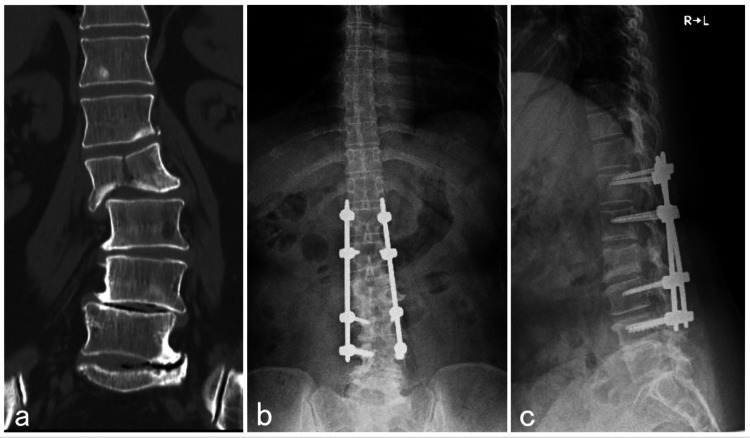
CT scan before surgery and plain radiograph after surgery The coronal CT scan shows a vertical fracture of the L3 vertebral body (a). Postoperative anteroposterior (b) and lateral (c) radiographs show pedicle screws were inserted from L1 to L5.
